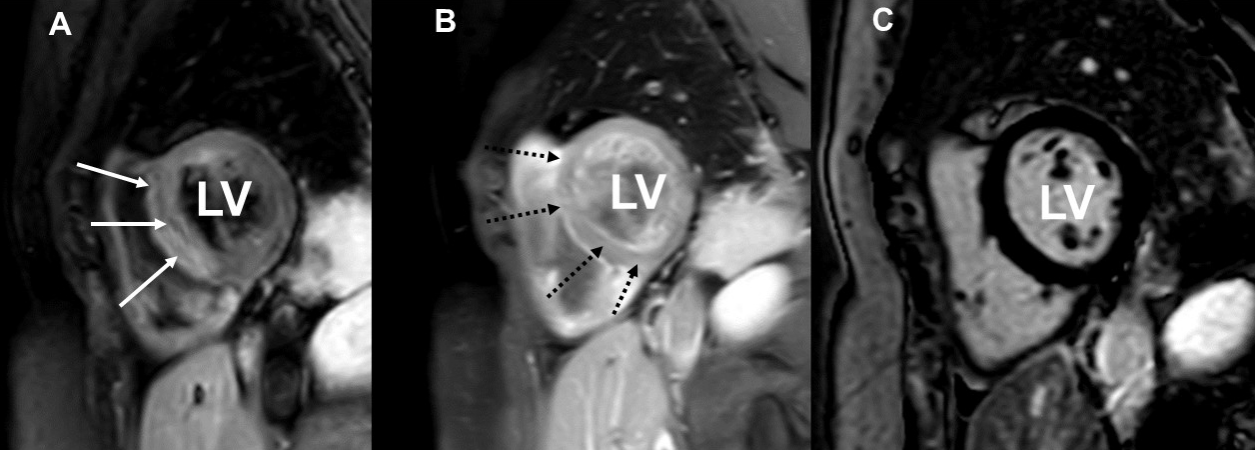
The three-year follow-up cardiac MRI of a 41-year-old-woman with systemic sclerosis demonstrated persistent regional high T2 signal intensity at mid anteroseptal and inferoseptal segments of the left ventricle (A: white arrows), patchy mid wall non-ischemic pattern on early gadolinium enhancement sequence (B: dashed arrows), no abnormal signal intensity of myocardium on late gadolinium enhancement (C), and abnormal high T2 mapping value of left ventricular myocardium on 1.5 Tesla MRI (56.5 ± 7.18 milliseconds) (D: black arrows). (LV: Left ventricle).
